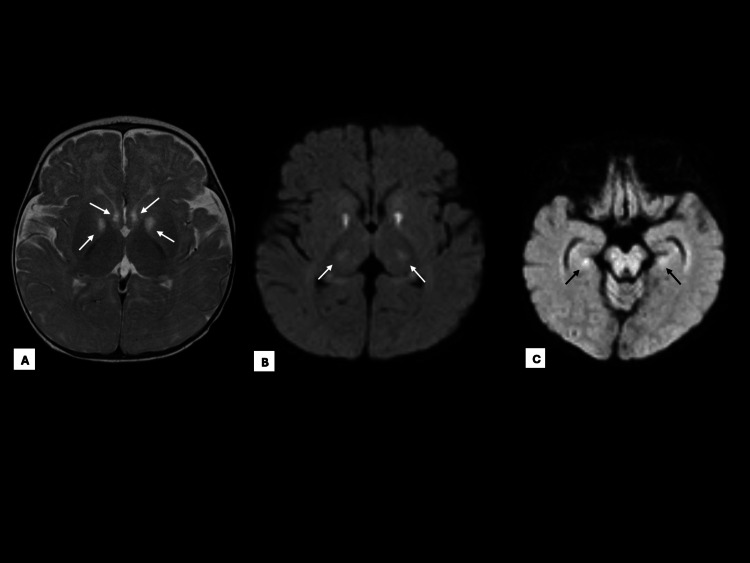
Readout segmentation of long variable echo-trains (RESOLVE) axial T2-diffusion-weighted MRI showing relatively bilateral and symmetric diffusion restriction within the basal ganglia; caudate nucleus and globus pallidus (A), bilateral thalami (B), and hippocampi (C).
